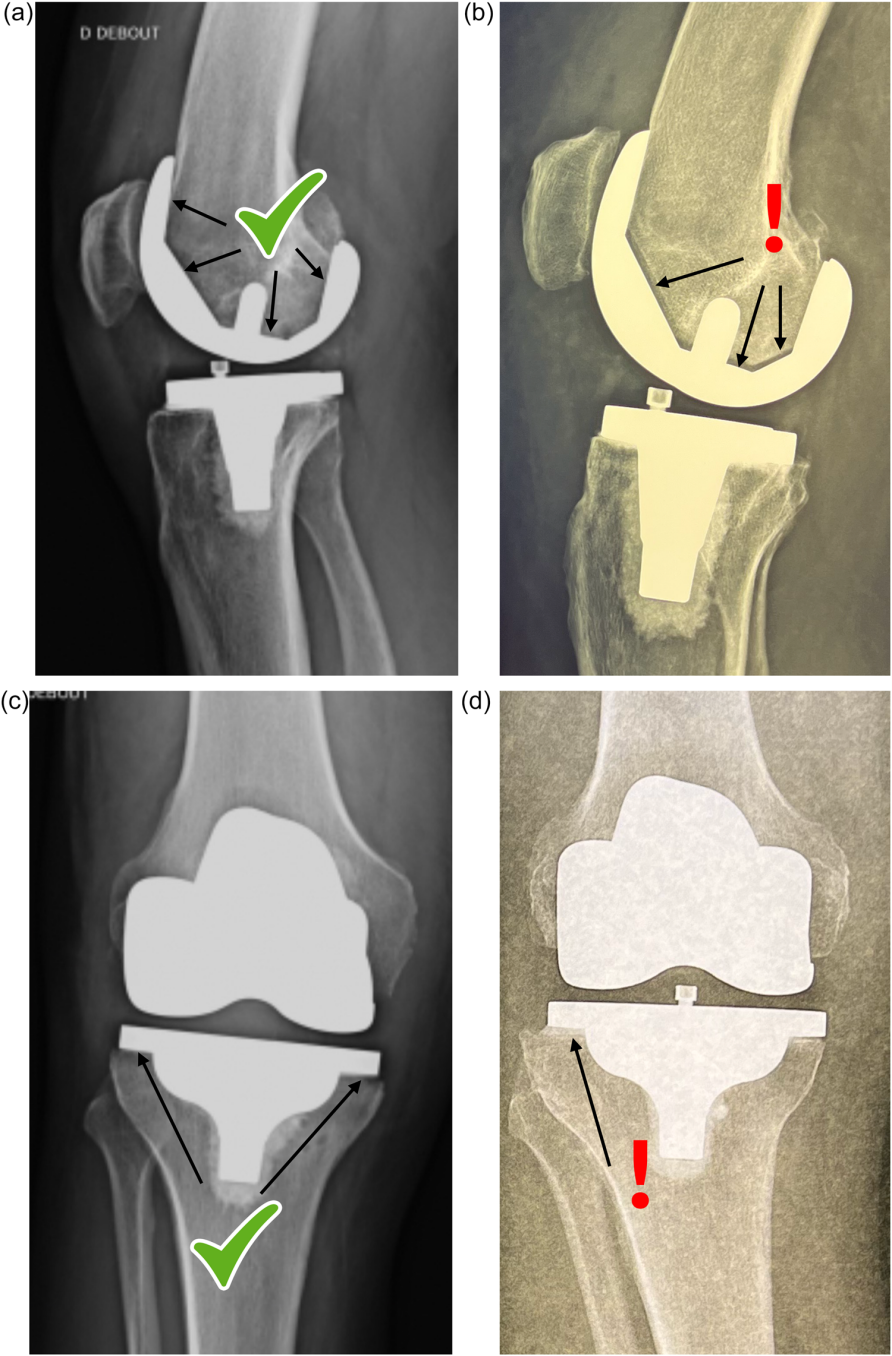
Radiographic assessment of adequate versus imperfect implant position. (a) Adequately positioned femoral component: No visible gaps between the distal femoral resection and femoral component on a sagittal view radiograph. (b) Imperfectly positioned femoral component: Visible gaps between the distal femoral resection and femoral component on a sagittal view radiograph. (c) Adequately positioned tibial baseplate: Uniform cement distribution between the proximal tibial resection and tibial baseplate component on a frontal view radiograph. (d) Imperfectly positioned tibial baseplate: Nonuniform cement distribution between the proximal tibial resection and tibial baseplate on a frontal view radiograph.
